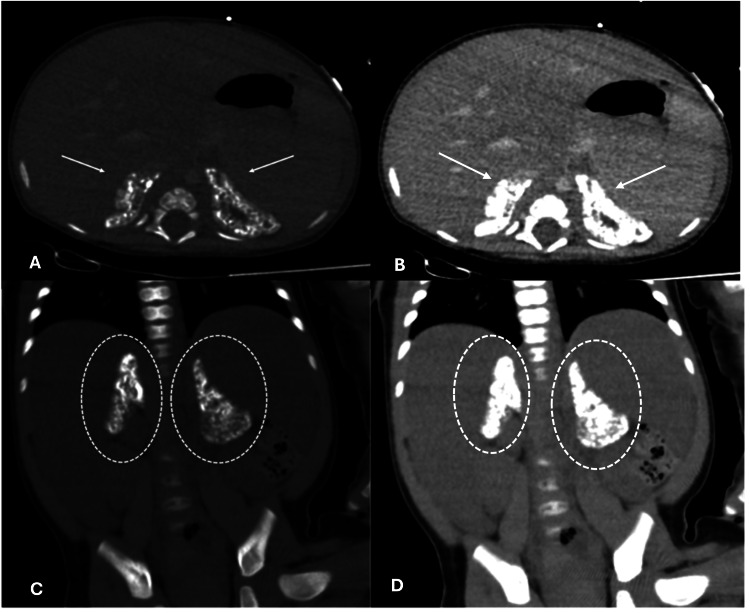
Selected contrast-enhanced abdominal computed tomography images displayed in soft-tissue and bone windows, in axial (A,B) and coronal (C,D) planes. Arrows and circles indicate the adrenal glands, which are enlarged and show punctate cortical calcifications while preserving their adreniform morphology
